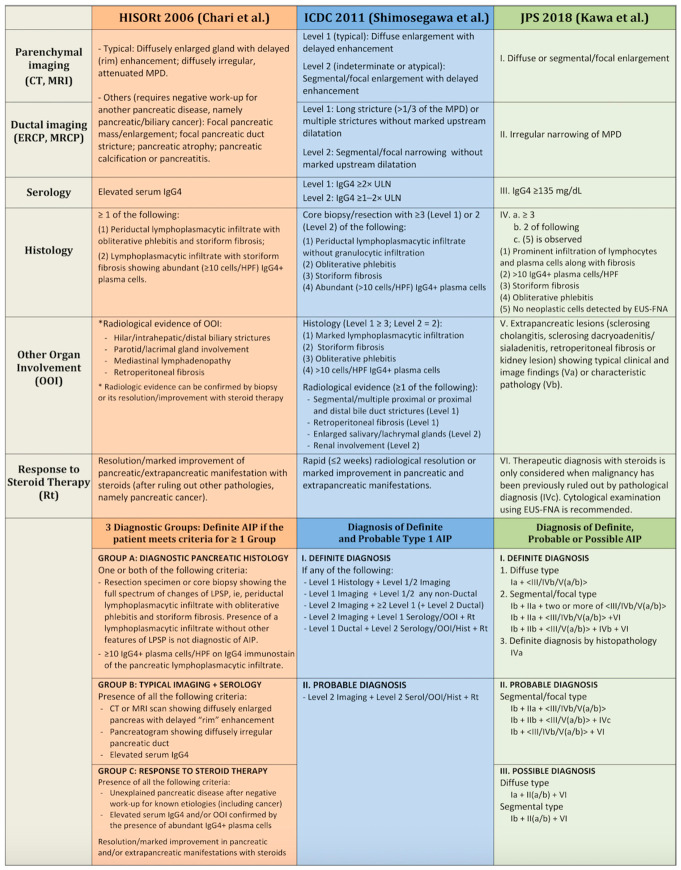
Summary of the main diagnostic criteria available for AIP: HISORt 2006, ICDC 2011 and JPS 2018. Adapted from Chari et al. [33], Shimosegawa et al. [34] and Kawa et al. [35]. MPD = main pancreatic duct; ULN = upper limit of normal value; LPSP = lymphoplasmacytic sclerosing pancreatitis; EUS-FNA = endoscopic ultrasound-guided fine-needle aspiration.
